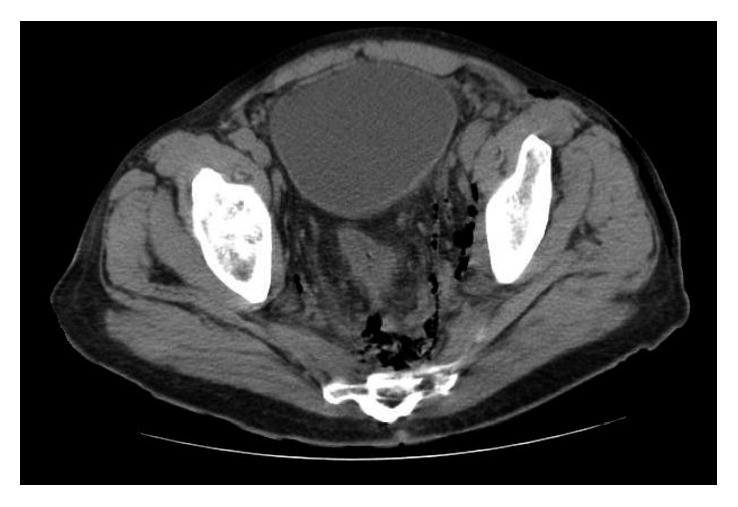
Axial CT image showing gas around the left rectum to the sacrum and ascending anterior along the left internal pelvic sidewall.
